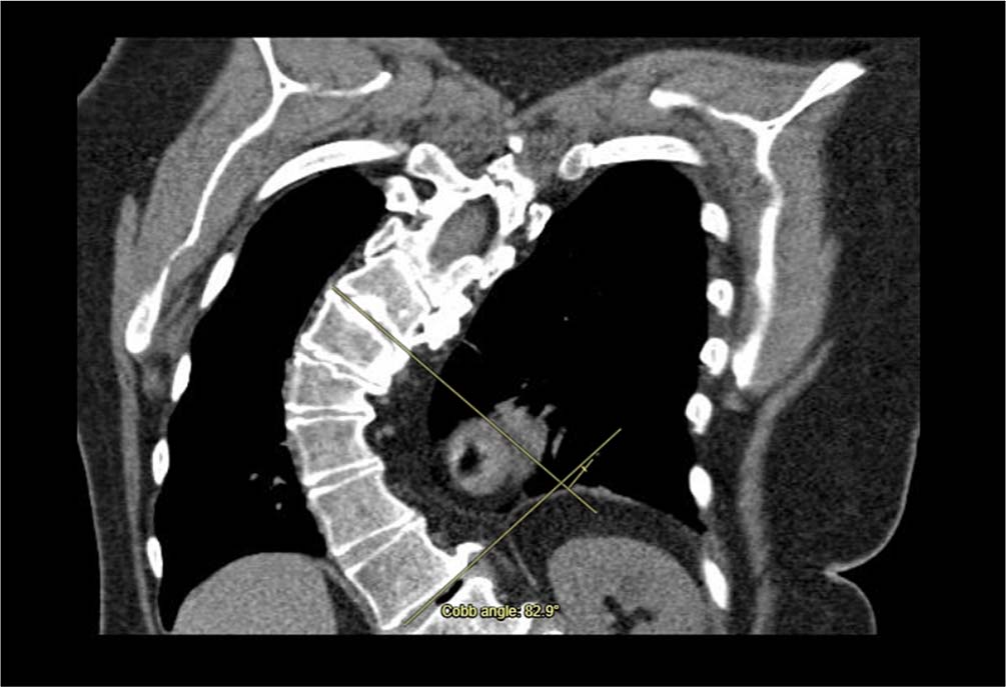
Coronal computed tomography (CT) with measurement of the cobb angle.
